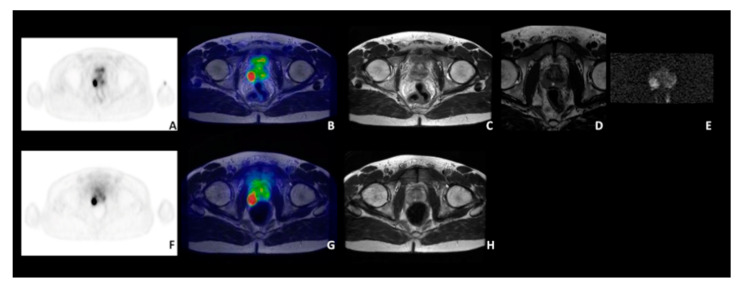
A 53 years-old patient with biopsy-proven PCa (pt n. 10), Gleason score 9 (5 + 4) with a PSA level at diagnosis of 3.13 ng/mL. Concordant ^68^Ga-PSMA PET/MRI (top panel; (A): transaxial ^68^Ga-PSMA PET; (B): ^68^Ga-PSMA PET/MRI; (C): Axial T2-weighted sequence; (D): Axial T2-weighted small FOV; (E): DWI (b = 1400)) and ^68^Ga-DOTA-RM2 PET/MRI (bottom panel; (F): transaxial ^68^Ga-DOTA-RM2 PET; (G): ^68^Ga-DOTA-RM2 PET/MRI; (H): axial T2-weighted sequence).
